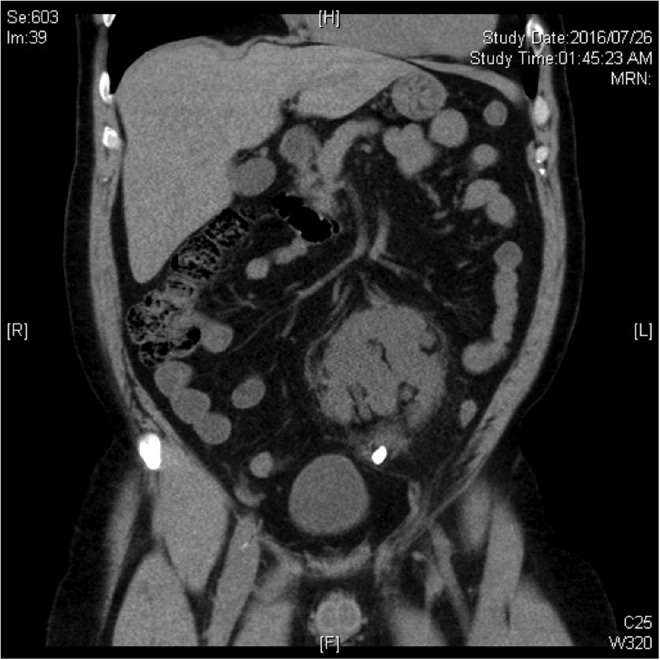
Coronal section of a noncontrast CT scan demonstrating a 1.1 cm proximal obstructing ureteric stone in a left pelvic kidney.
